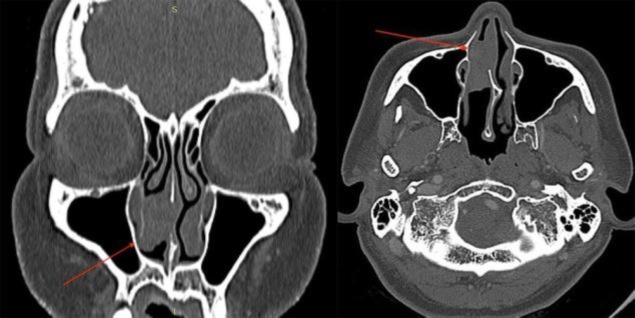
Figure showing the CT paranasal findings of a homogenous non-enhancing mass (red arrow) occupying the right nostril
